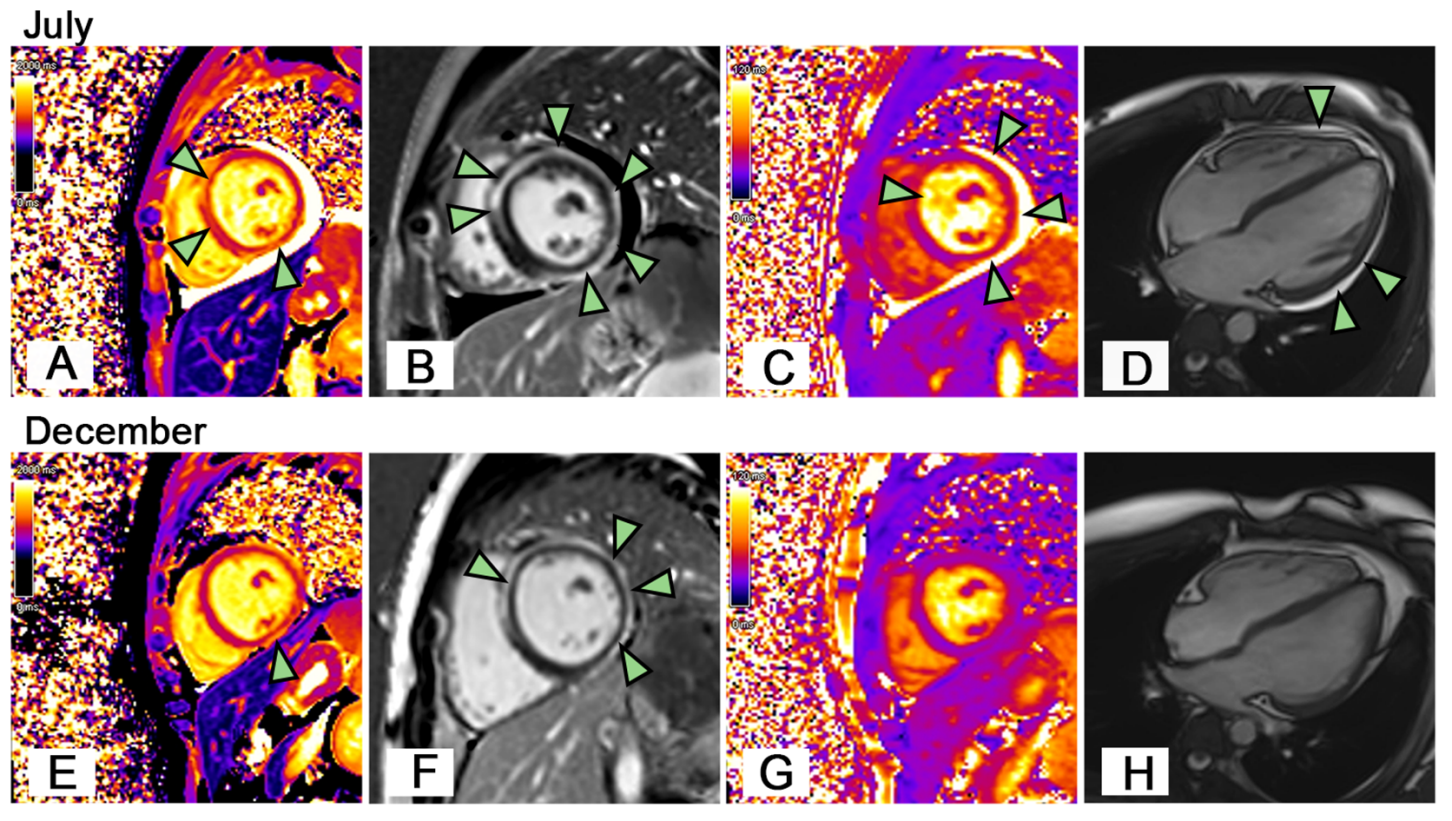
Cardiac Magnetic Resonance Imaging. On July (A) The patient’s short-axis view showed local increases in T1 values in the anterior septum and inferior wall of the left ventricle (arrowheads). (B) The patient’s LGE image showed delayed subepicardial enhancement of the left ventricle, including the anterior septum, anterior wall, lateral wall, posterior wall, and inferior wall (arrowheads). (C) The patient’s short-axis view showed increased T2 values in the left ventricular myocardium (edema), especially in the anterior septum, inferior, posterior, and lateral walls (arrowheads). (D) The patient had pericardial effusion (arrowheads). On December (E) The T1 value of the anterior septum of the left ventricle decreased to normal, while the local T1 value of the inferior wall was still increased (arrowhead). (F) LGE image showed the delayed enhancement of the left ventricular subepicardium persisted (arrowheads). (G) The overall left ventricular T2 value decreased to normal. (H) The pericardial effusion disappeared.
